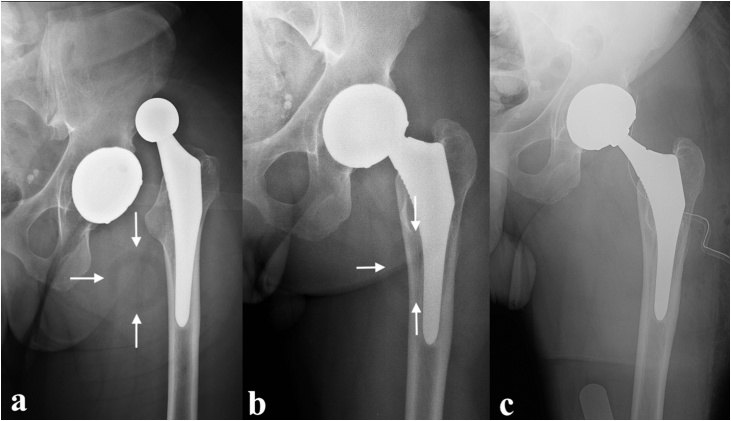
X-rays takenbefore (a) and after (b) closed reduction in our department (the arrows point to the “bubble sign”). Control radiograph after revision surgery (c).
